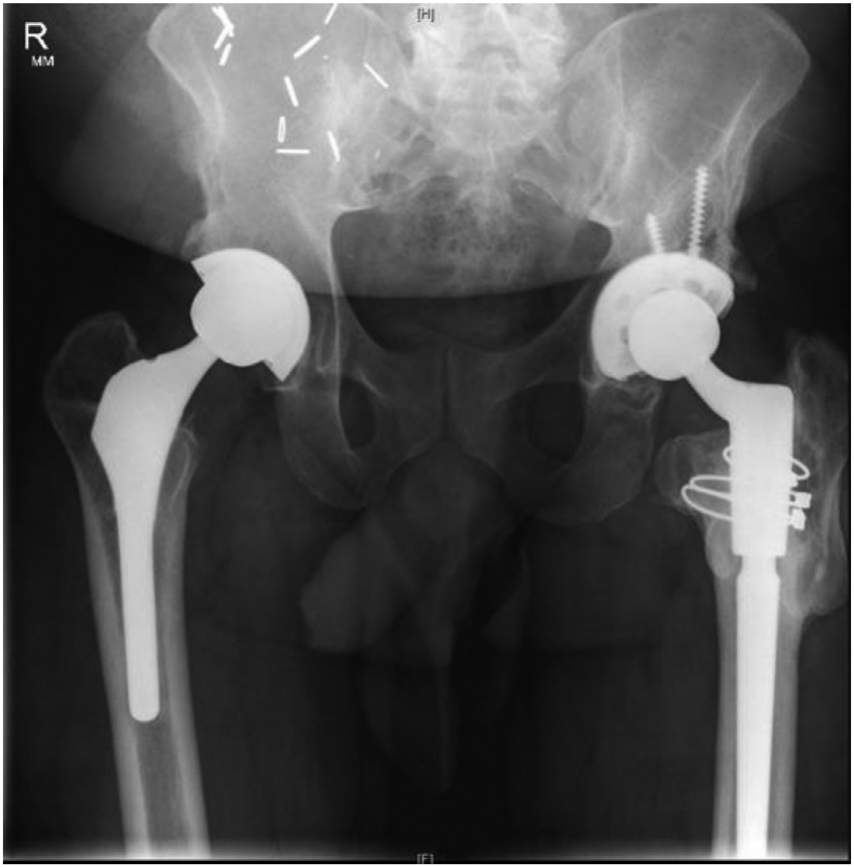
Initial pelvis radiographs for Patient 2-case 3. A 64-year-old man with a right metal-on-metal THA with slight eccentric positioning of the femoral head within the acetabulum. The metal inlay can be visualized on close inspection. A previous revision THA is visualized in the contralateral limb.
